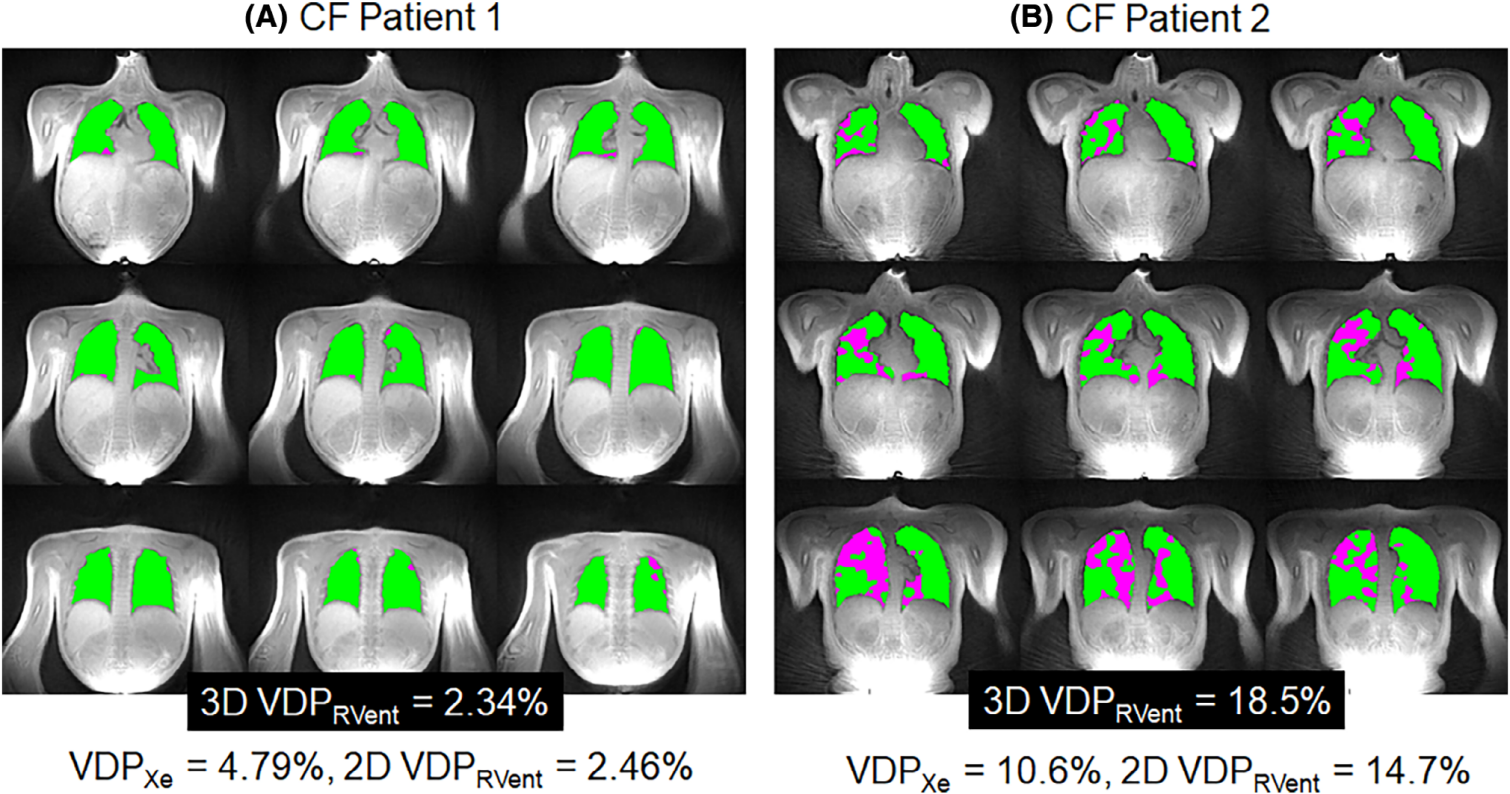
Ventilation defect maps derived from 3D UTE phase‐resolved functional lung (PREFUL) MRI regional ventilation (RVent) distributions for two representative pediatric CF patients with (A) low 3D RVent VDP (3D VDP_RVent_) and (B) high 3D VDP_RVent_. Hy ^129^Xe‐MRI derived VDP (VDP_Xe_) and 2D PREFUL MRI RVent VDP (2D VDP_RVent_) are shown for each CF patient. CF, cystic fibrosis; VDP, ventilation defect percentage.
